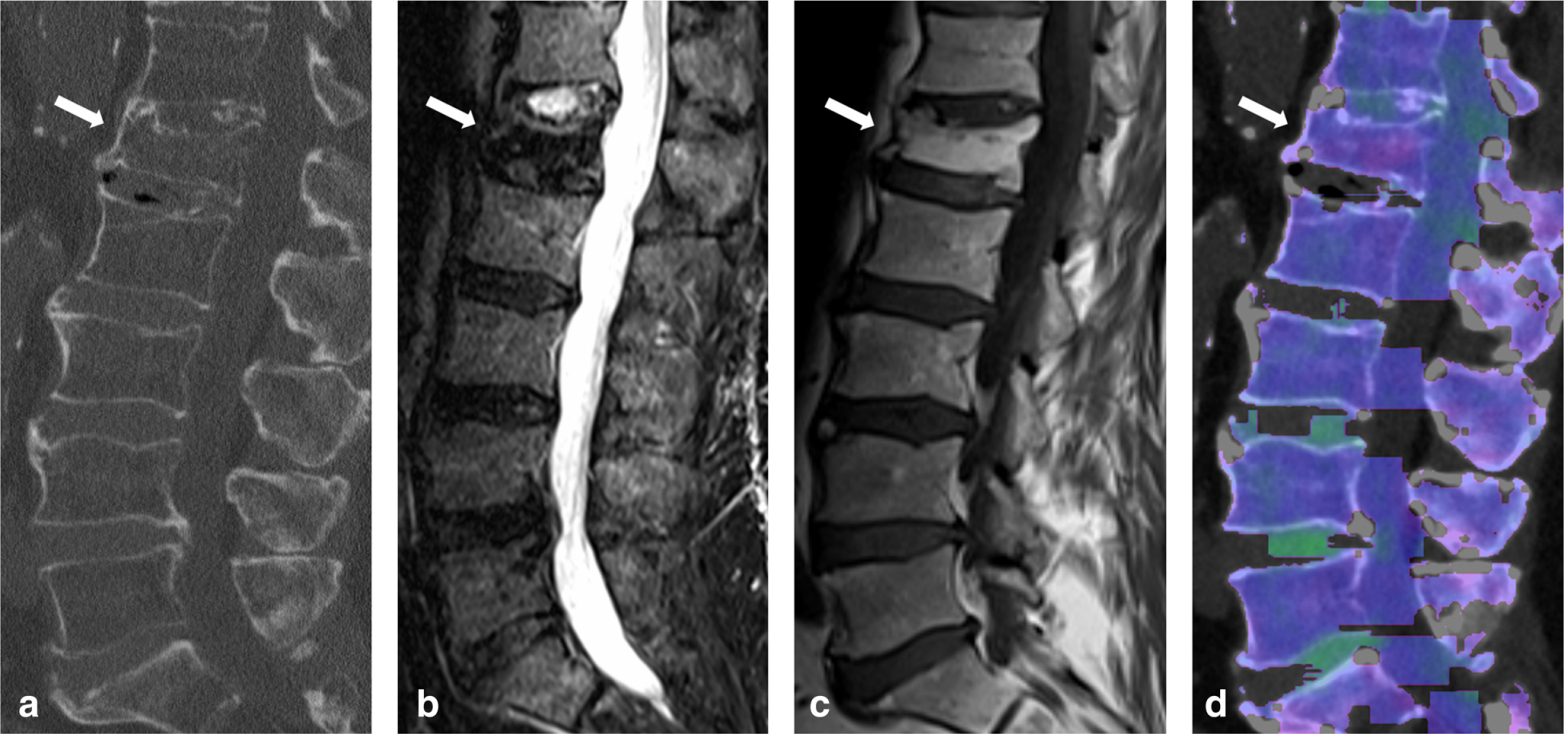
Seventy-one-year-old man presenting with spinal trauma after fall accident on a street. a Initially performed dual-energy computed tomography (DECT) showed a distinct compression fracture involving the superior endplate of L1 on sagittal grayscale DECT series. No further post-processing such as creation of color-coded virtual non-calcium (VNCa) reconstructions was performed in this patient in daily routine. b, c Five days later, additionally performed magnetic resonance imaging (MRI) demonstrated decreased signal intensity of L1 on sagittal turbo inversion recovery magnitude (TIRM)-MR series and an increase of signal intensity on sagittal T1-weighted spin-echo MRI (*arrows*). Therefore, the fracture was considered as old with complete fatty degeneration of bone marrow. d VNCa images (only reconstructed for the purpose of this study) show no evidence of bone marrow edema (BME) in L1 (*arrowhead*) (score, 0; for all readers). Furthermore, a decrease of signal intensity on VNCa images (displayed as violet area) indicates fatty degeneration of bone marrow in L1 (*arrowhead*). It is conceivable that initial reconstruction of color-coded VNCa reconstructions in daily routine would have facilitated the correct diagnosis of an old vertebral fracture
